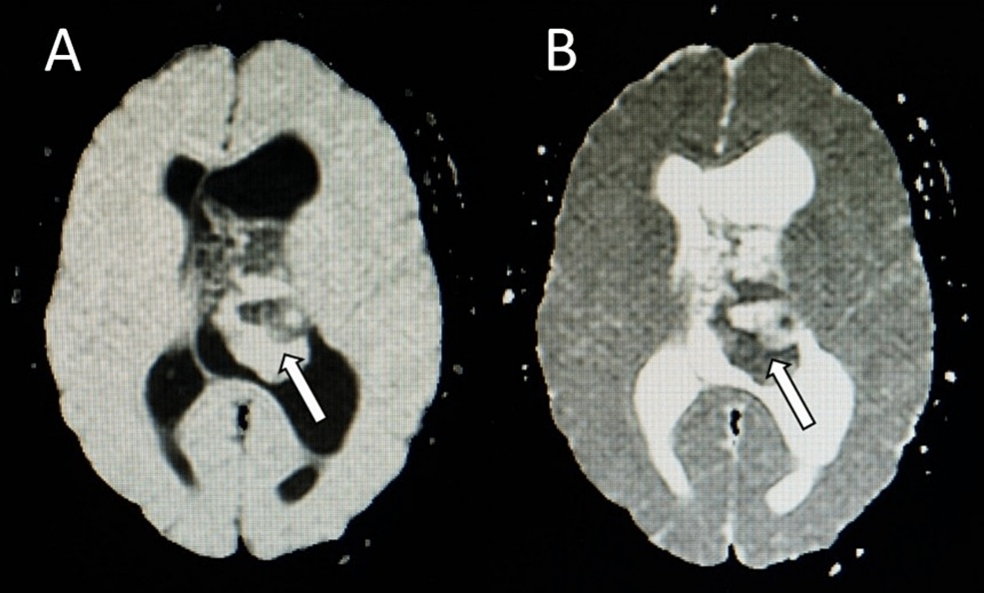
Axial DWI of the brain. (A) DWI and (B) enhanced or exponential DWI shows diffusion restriction of the solid portion of the mass (arrows), suggesting high cellularity of the mass. DWI: diffusion-weighted imaging
